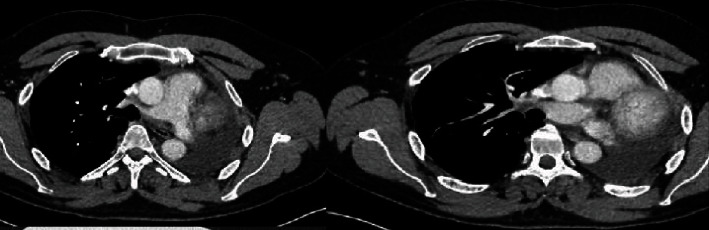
CT scan after left pneumonectomy.
